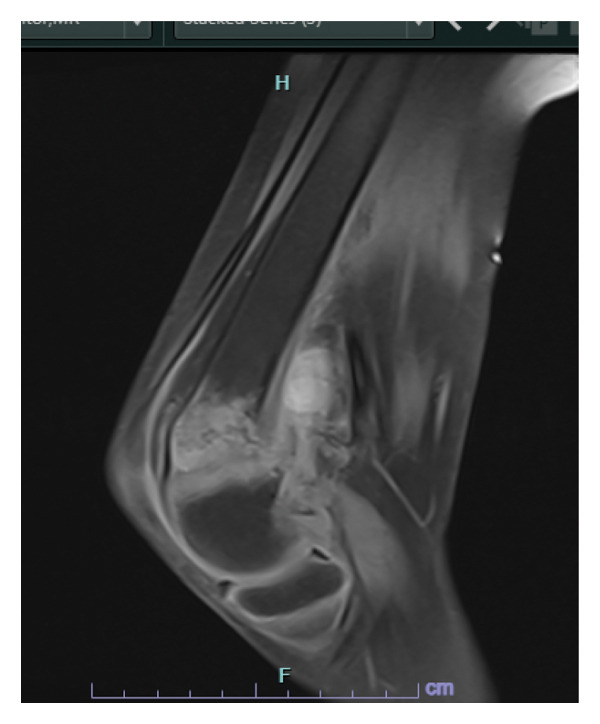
Magnetic resonance imaging of the right knee showed a transverse distal femoral fracture associated with periosteal elevation and intermediate callus formation.
